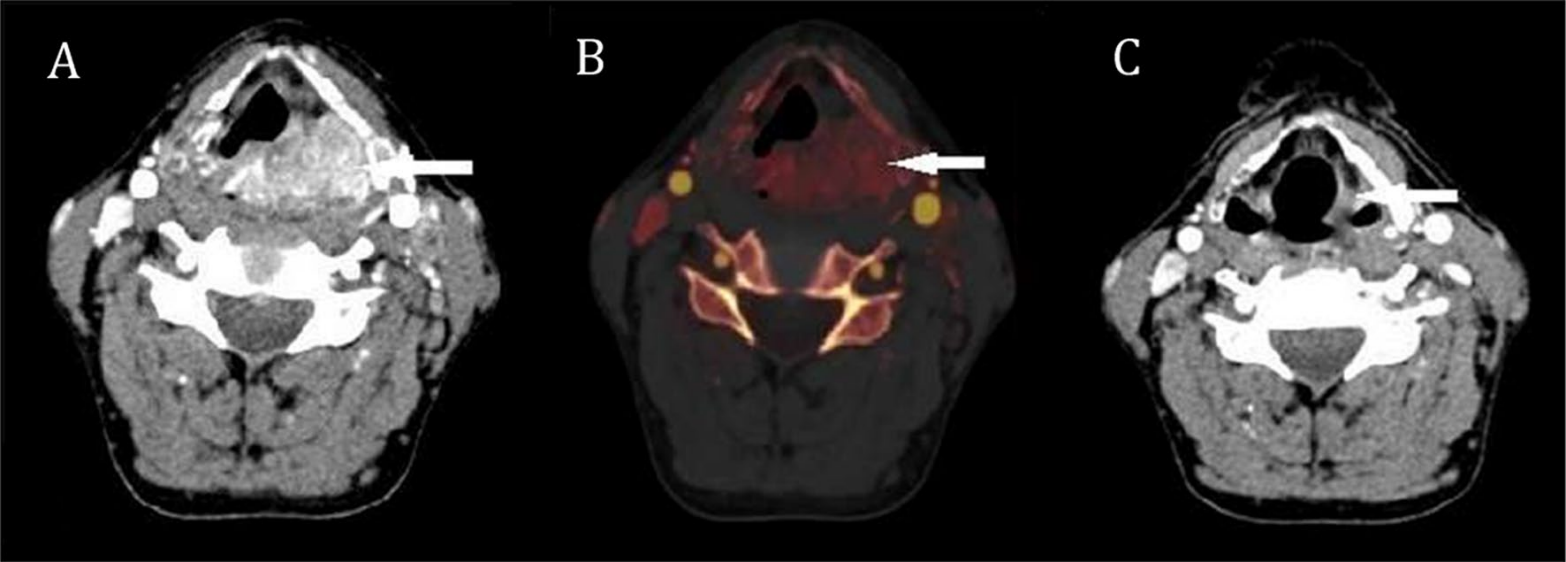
A representative hypopharyngeal cancer patient showed partial remission after neoadjuvant chemotherapy. (A) Axial CT image of arterial phase before chemotherapy showed an obviously enhanced tumor at left piriform fossa extending to the posterior pharynx wall and postcricoid region. (B) The iodine map of arterial phase before chemotherapy, the red color showed obvious iodine-related attenuation (IRA_mean_ 48.8HU, IRA_max_ 76.5HU) in the tumor. (C) Followup CT image after chemotherapy, the lesion at the left piriform fossa was significantly smaller.
